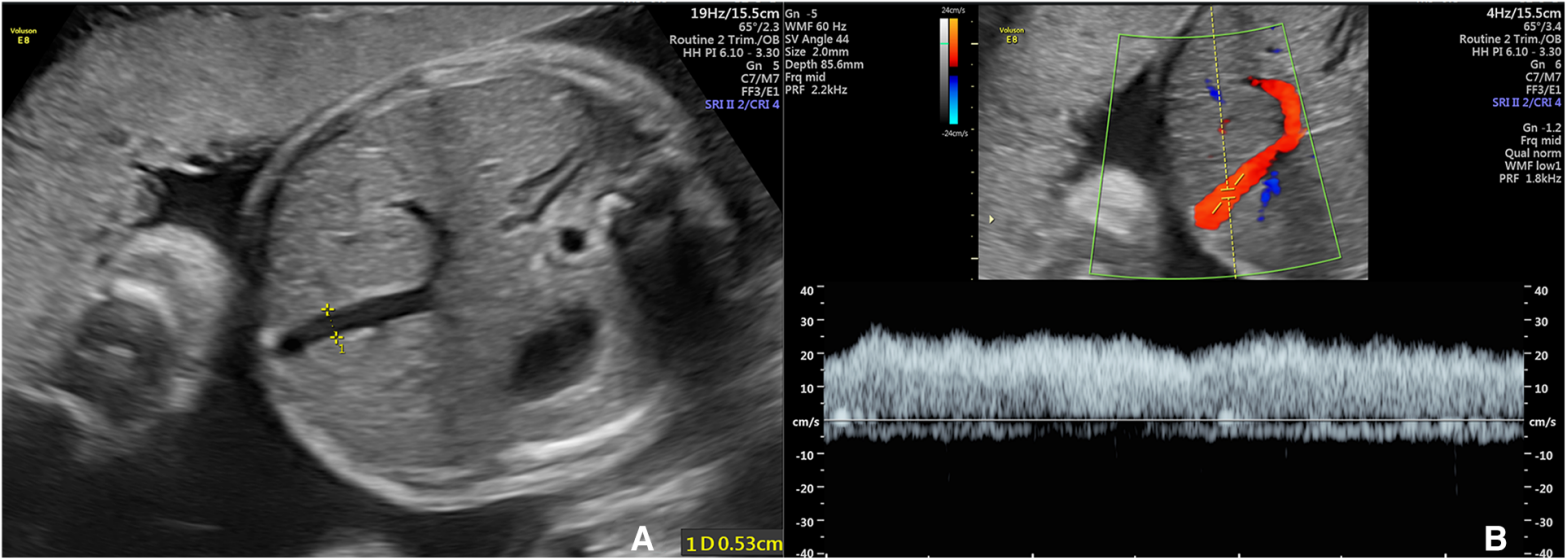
The ultrasonic measurment of fetal umbilical vein. (A) Velocity of umbilical venous blood flow (Vuv); (B) Diameter of umbilical venous blood flow (Duv).
